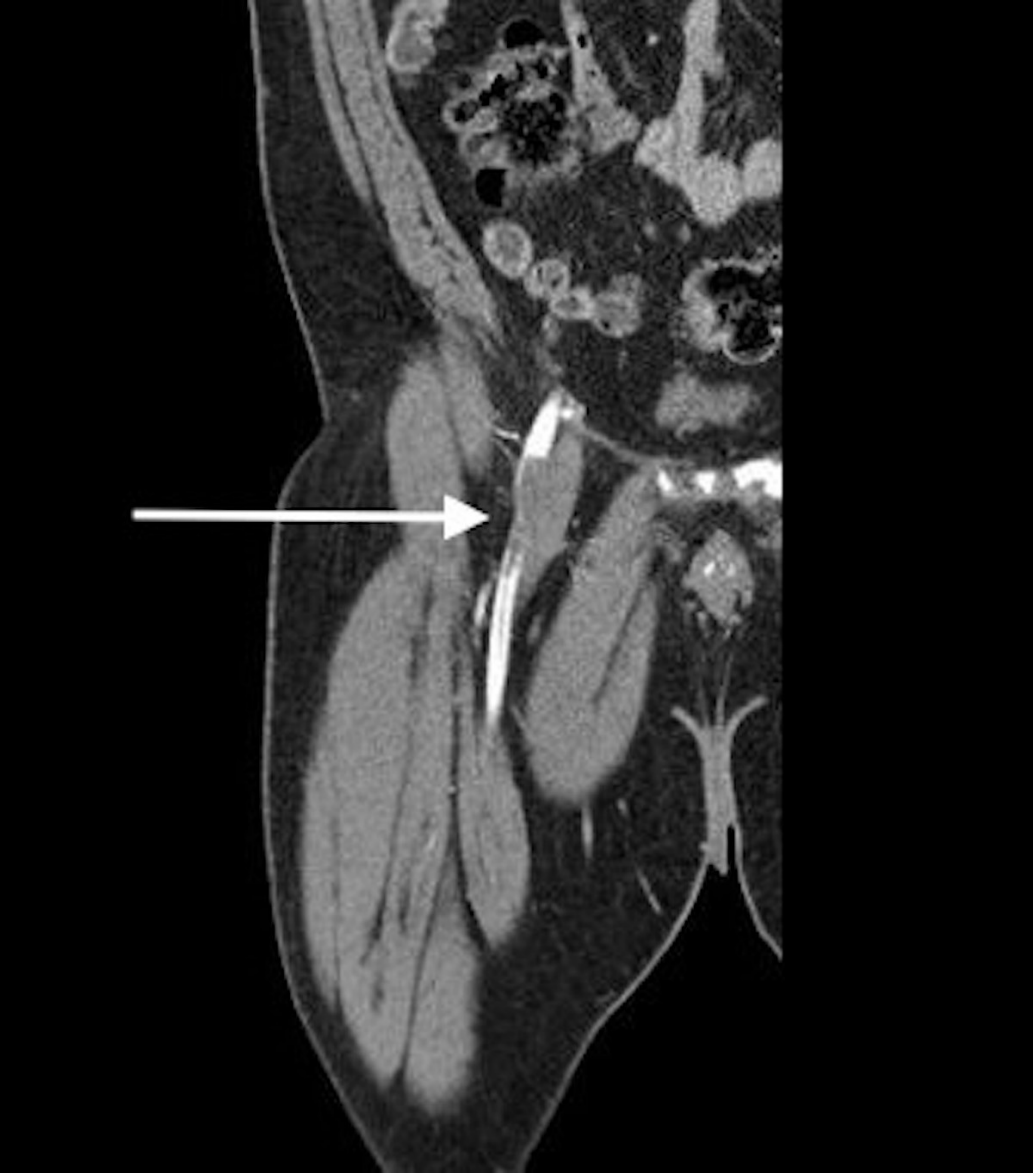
Computed tomography with contrast (frontal plane) of left lower extremity demonstrating occlusion of the common femoral artery.
